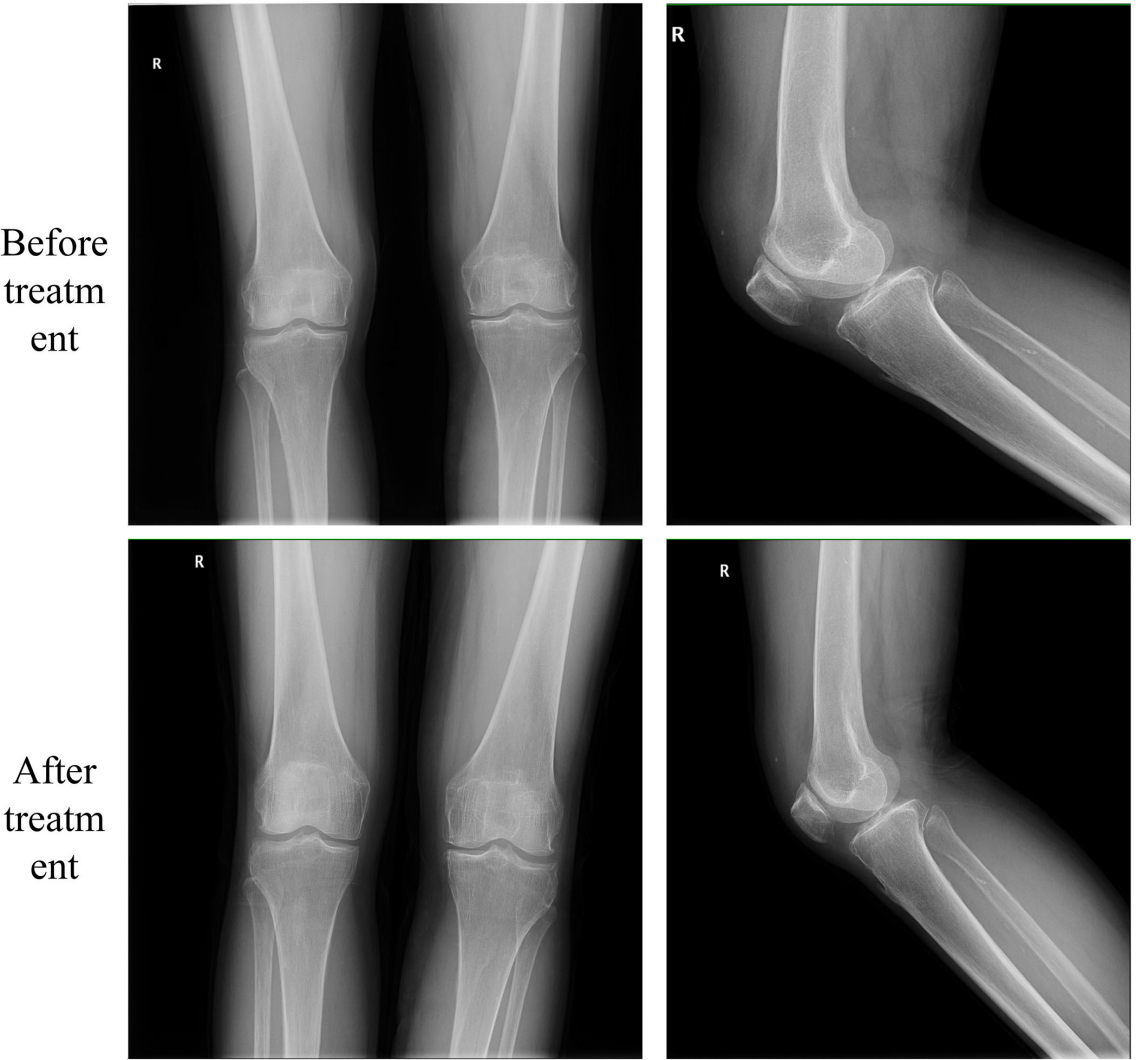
X-ray before and after treatment. X-rays of the knee before treatment were suggestive of knee osteoarthritis (Grade III on the K–L grading). K–L, Kellgren–Lawrence.
